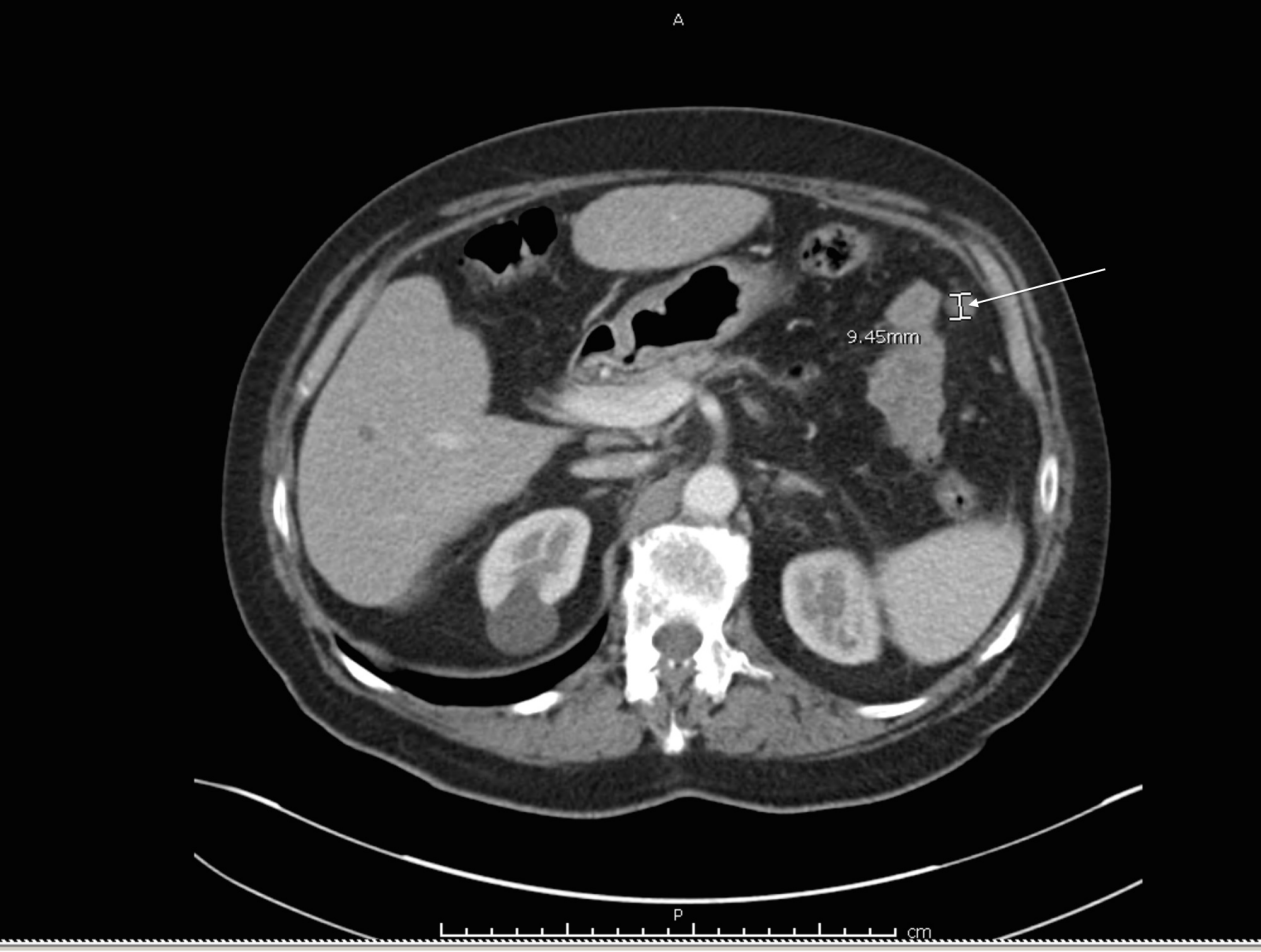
Large peritoneal nodule CT scan study was done with intravenous contrast five months after presentation highlighting an enlarging peritoneal nodule. CT: computed tomography
